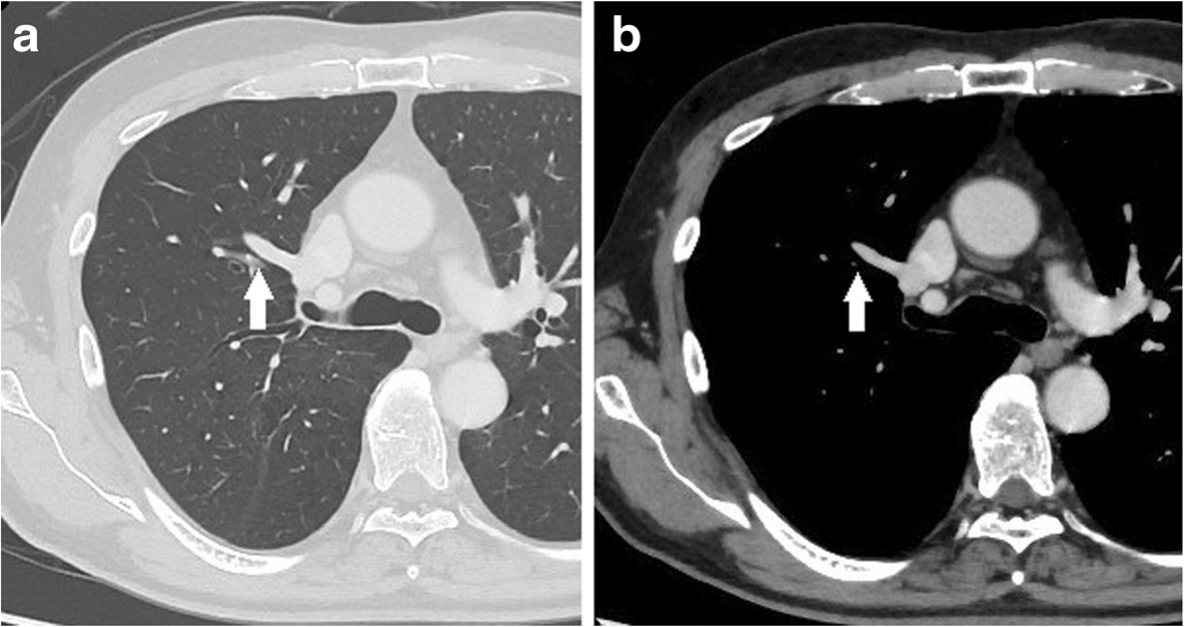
Computed tomography image. The chest computed tomography image shows an enhanced anomalous vein that continues from the superior vena cava (*arrow*). a The mediastinal window. b The lung window
